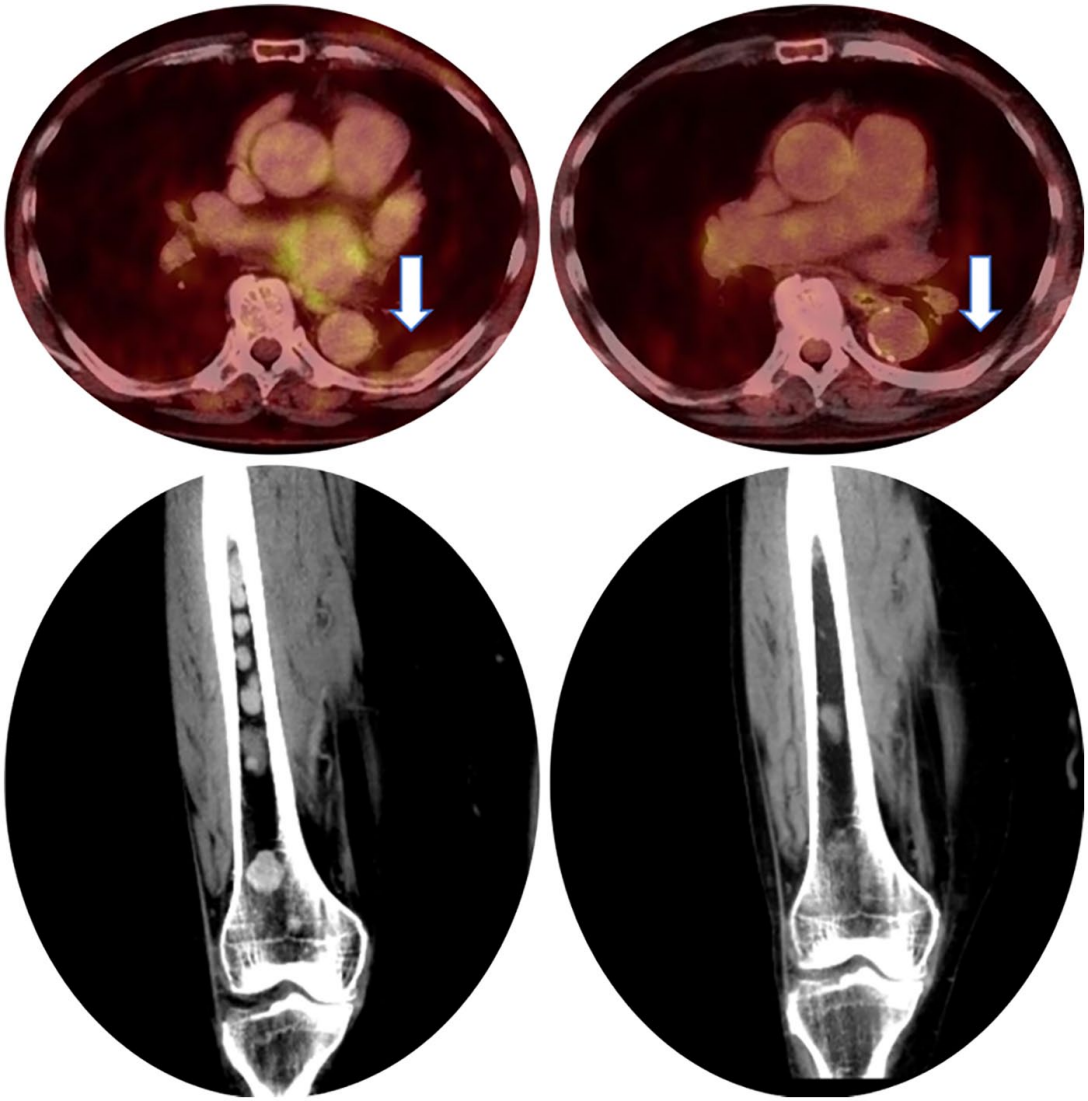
Left: 18F-FDG-PET/CT scan in August 2018 showed pleural extramedullary disease (EMD) and focal bone lesions of the right femur. “Pom-PAD-Dara” (pomalidomide, bortezomib, doxorubicin, dexamethasone, daratumumab) had been initiated 2 days prior to imaging and therefore, we observed a significant reduction of EMD metabolism. Right: 18F-FDG-PET/CT scan in August 2019 demonstrated therapy response after nine cycles of “Pom-PAD-Dara”.
